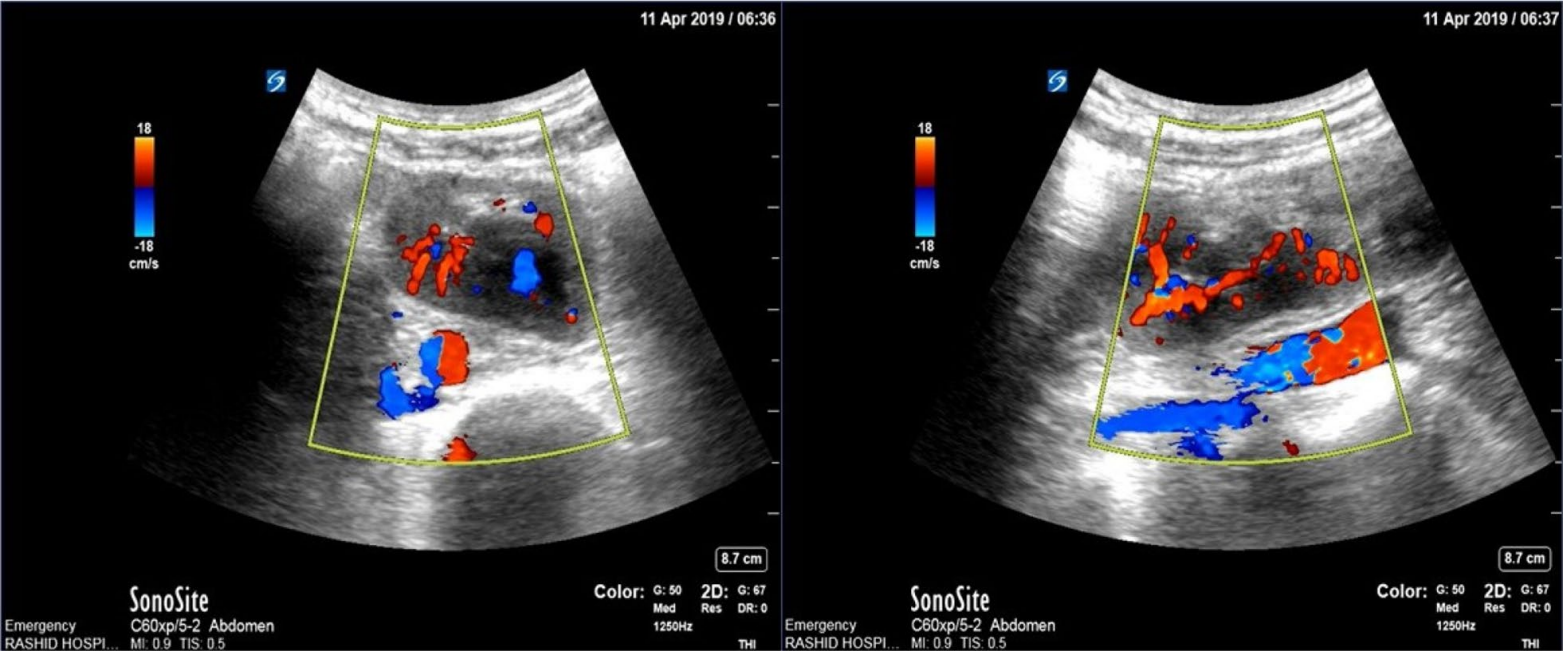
The color Doppler of great value by demonstrating a normal vascular architecture that is compatible with renal vessels
